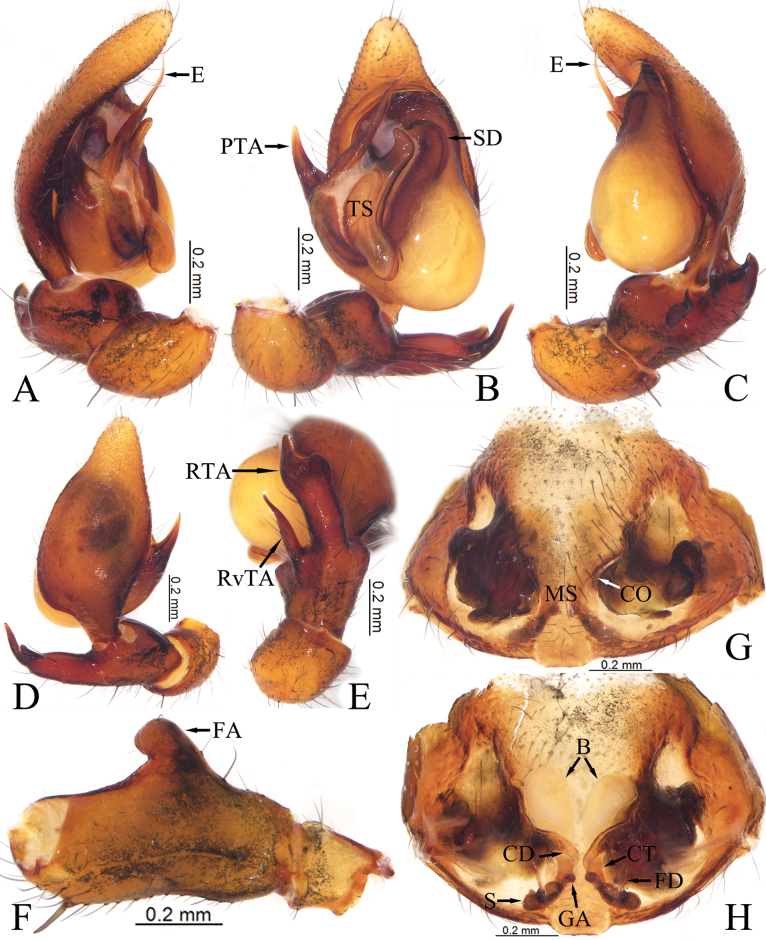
Copulatory organs of *Mutatusgelao* sp. nov. A male left palp, prolateral view B same, ventral view C same, retrolateral view D same, dorsal view ERTA, retrolateral view F femur, prolateral view G epigyne, ventral view H same, dorsal view. Abbreviations: E—embolus; FA—femoral apophysis; PTA—prolateral tegular apophysis; RTA—retrolateral tibial apophysis; RvTA—retroventral tibial apophysis; SD—sperm duct; TS—tegular sheet; B—bursa; CD—copulatory duct; CO—copulatory opening; CT—connecting tube; FD—fertilization duct; GA—glandular appendage; MS—median septum; S—spermathecae.
